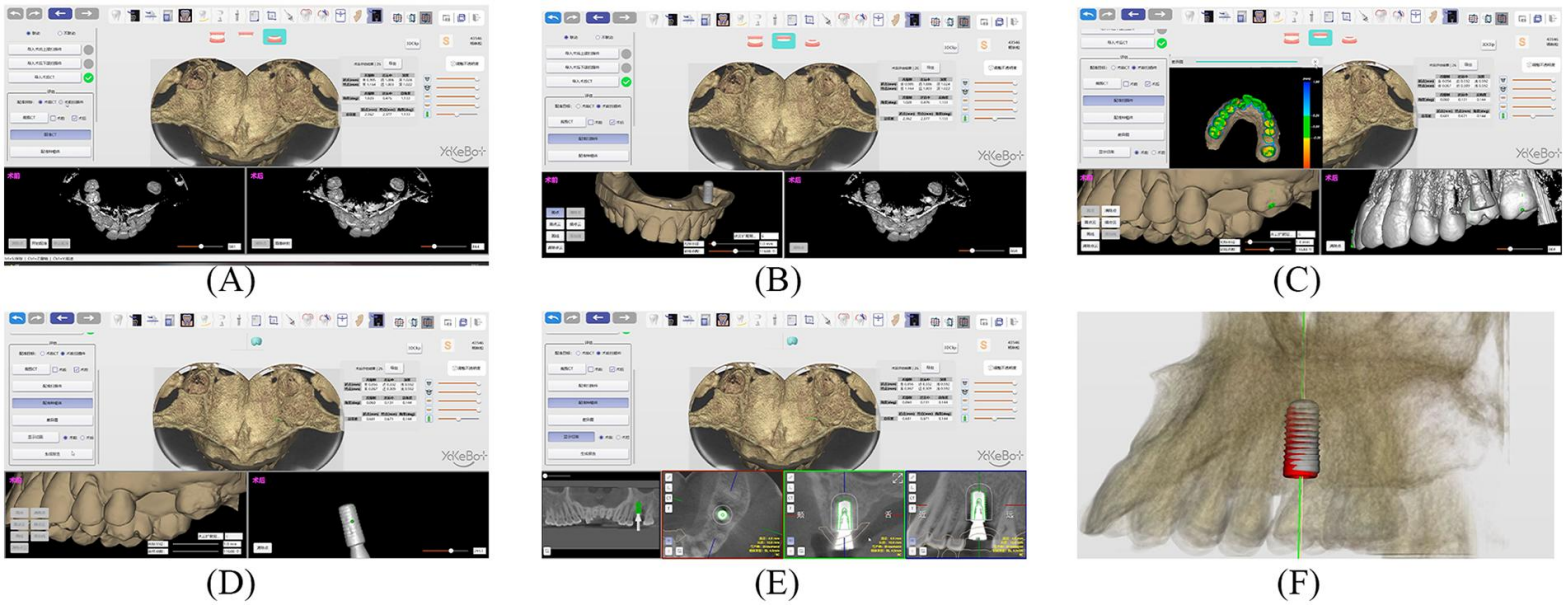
(A,B) Postoperative and preoperative CBCTs with virtual planning were imported into dentalNavi. (C,D) Registration of scans and implants. (E,F) The 3D view of the preoperative plan compared with postoperative CT.
